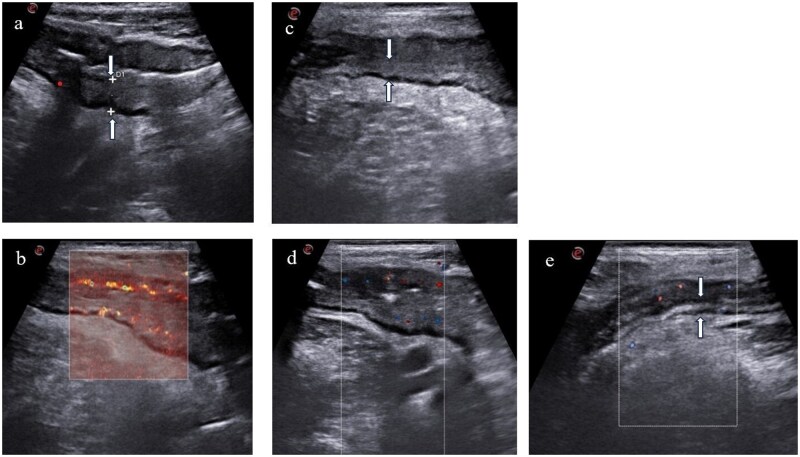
(A and B) Inflammation in the terminal ileum seen on intestinal ultrasound consistent with severely active Crohn’s disease and characterized by increased bowel wall thickness to 10 mm (white arrows, panel A) and severe grade of vascularization (Limberg score 4, panel B) before anti-TNF⍺ initiation. (C and D) After three months of biological therapy, improvement of bowel wall thickness of the terminal ileum (6 mm, white arrows, panel C) with mild grade of vascularization (Limberg score 2, panel D) (ΔBWT [4 mm] and Limberg score improvement [2 points] applied to this case). (E) At 12 months of biological therapy, further improvement of bowel wall thickness of the terminal ileum (4 mm, white arrows) with persistent mild grade of vascularization (Limberg score 2).
